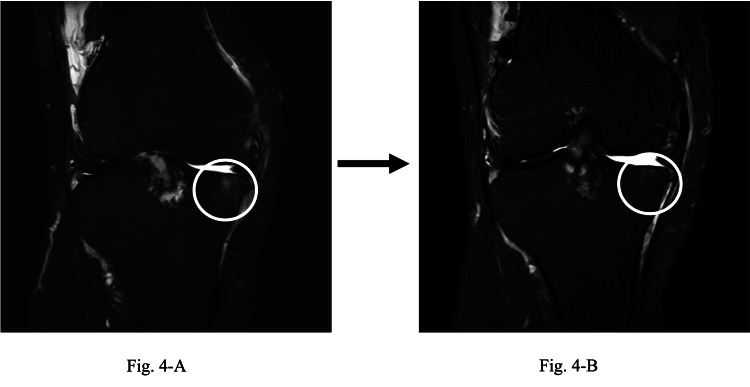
Comparison of BML size MRI before and after intervention. Change in BMLs. The BMLs, which were initially graded 2 (A), were reduced to grade 1 after 12 months (B). BML, bone marrow lesion; MRI, magnetic resonance imaging
